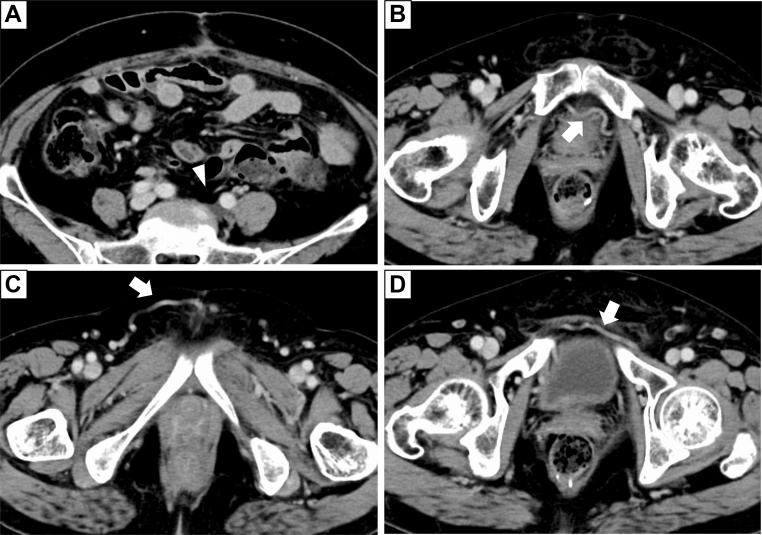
Postoperative contrast-enhanced computed tomography (CT) at 3 months after surgery. (A) Left common iliac vein (CIV) and left common iliac lymph node were completely resected, and there was no evidence of recurrence (*white arrowhead*). (B) Development of collateral veins from left to right within the vesical venous plexus was observed (*white arrow*). (C) Development of collateral veins from the superficial external pudendal vein was observed (*white arrow*). (D) Development of the pubic vein was observed (*white arrow*).
